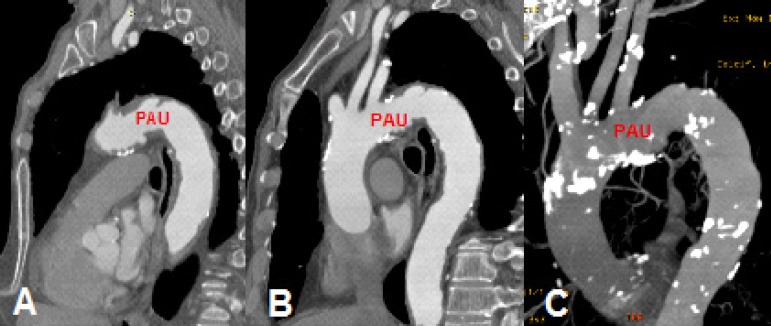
Case 1: computed tomography (CT) angiography of penetrating aortic
